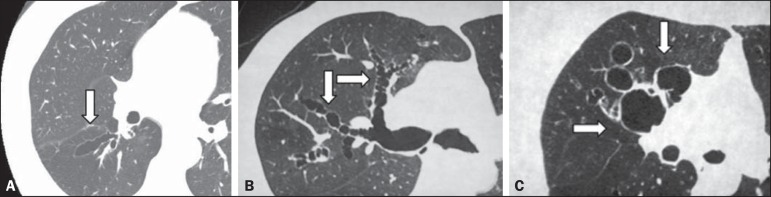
CT of the chest in patients with different patterns of bronchiectasis
